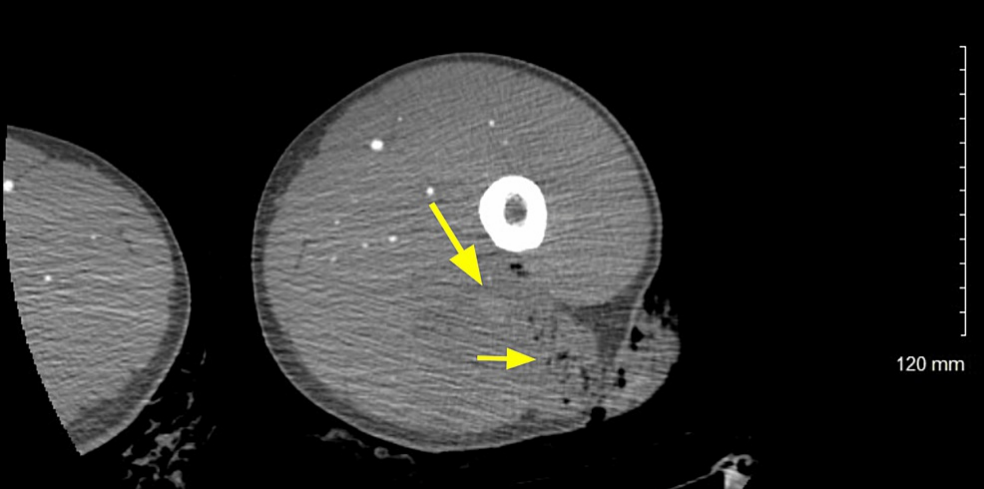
Axial CT angiogram The image shows air in the muscle (small arrow) and active extravasation of contrast (large arrow).
